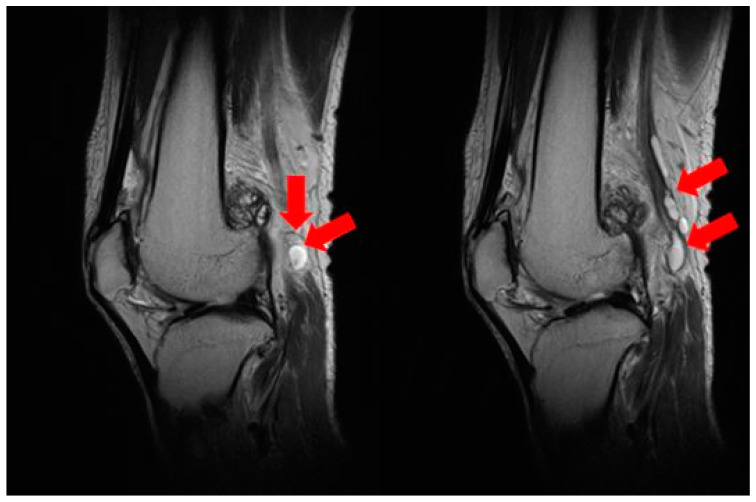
A preoperative knee T2-weighted sagittal MRI showing multiple ganglion cysts (red arrow).
